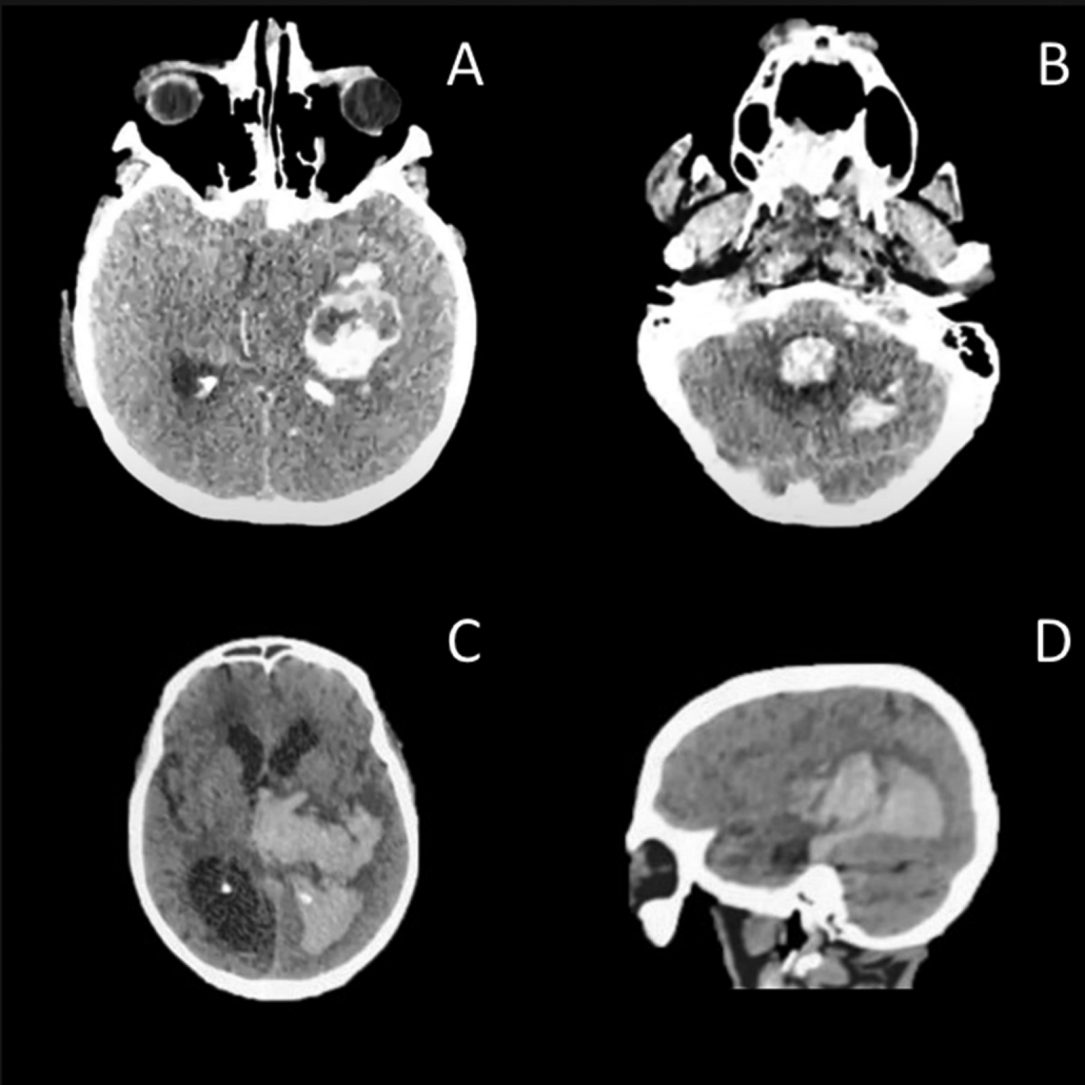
Intraparenchymal hemorrhages. (A-B) Patient 1: 66 years-old male presenting with decreased level of consciousness. Axial non-contrast CT images (
